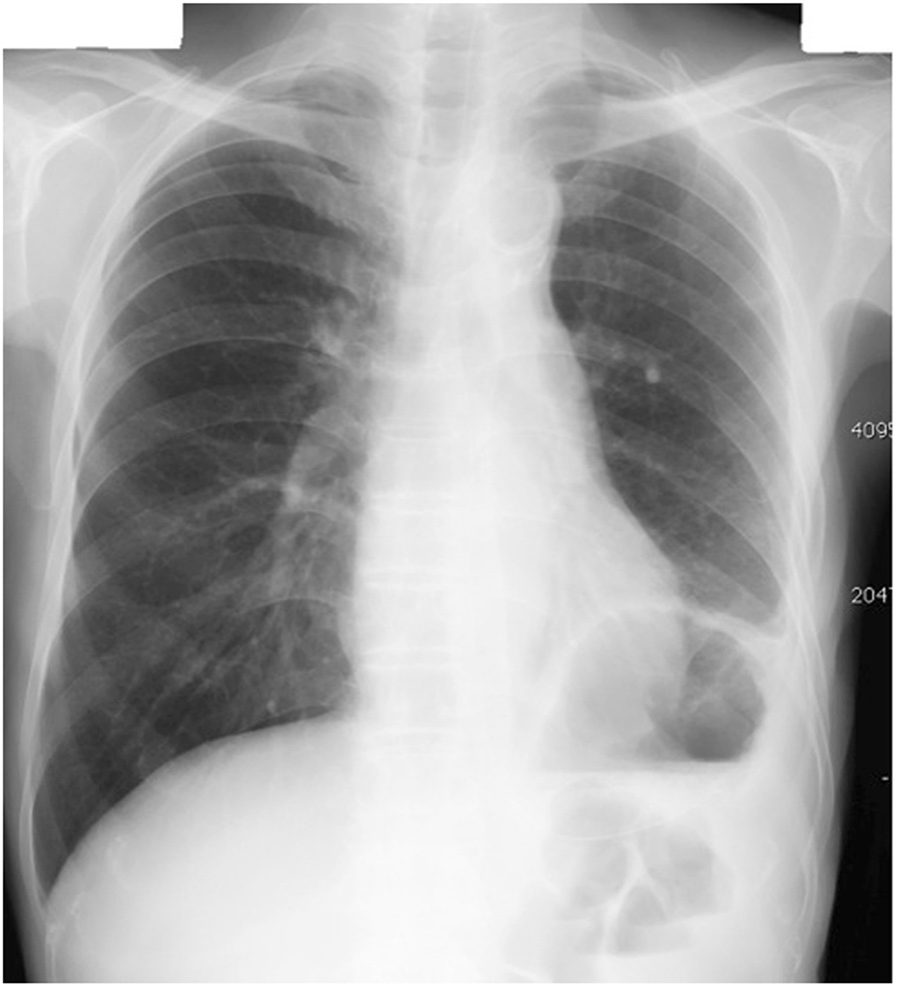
A preoperative chest roentgenogram demonstrating intrathoracic air–fluid level in the left chest.
